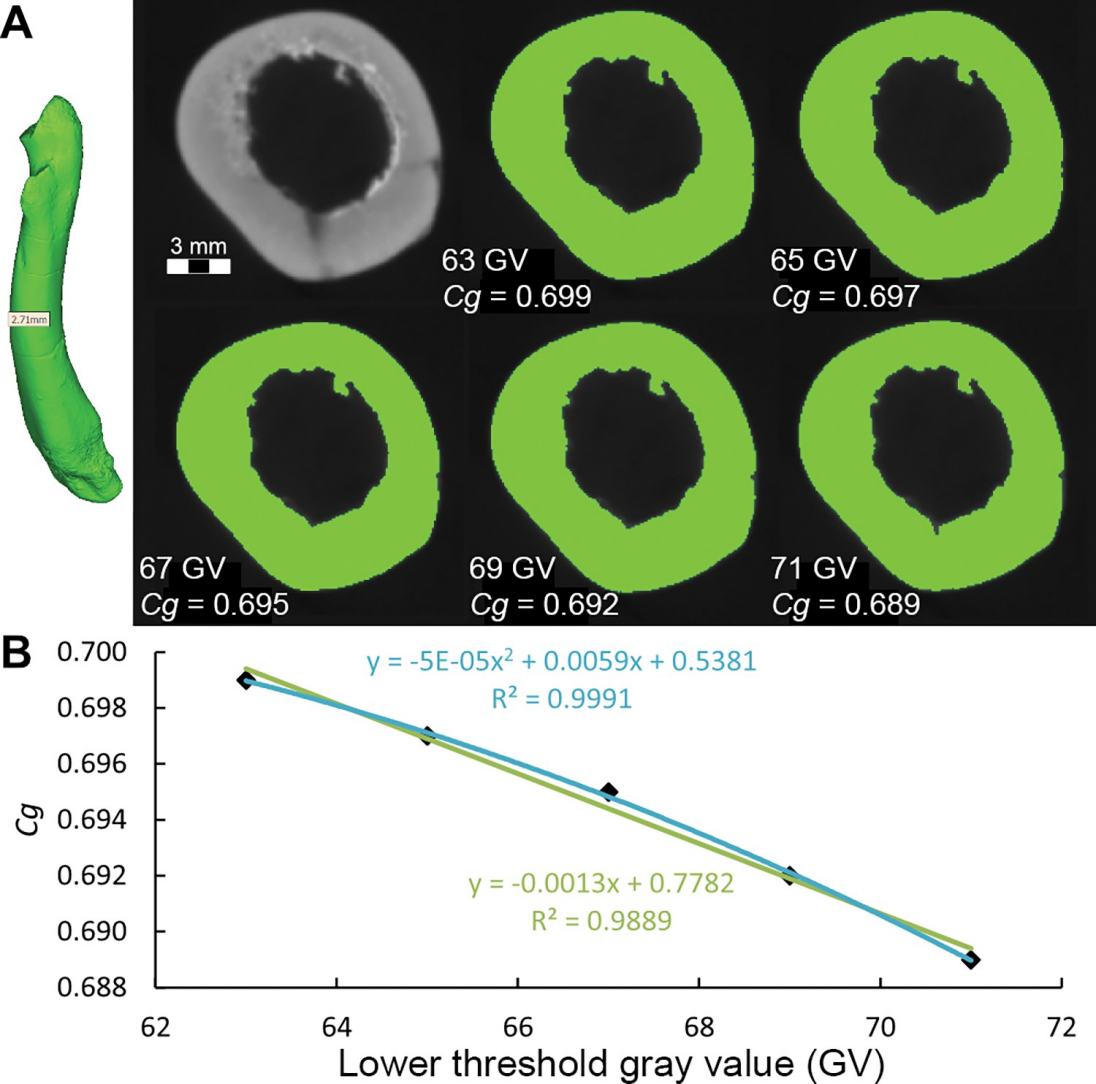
Bone compactness derived from CT scan of a juvenile femur of cf. *Spinosaurus aegyptiacus* (CMN 50382). (A) 3-D rendering and midshaft cross section generated from a CT scan, with binarized images (green) differing in their lower threshold gray-value setting. (B) Plot showing linear change of about 10% in *Cg* over the threshold range.
